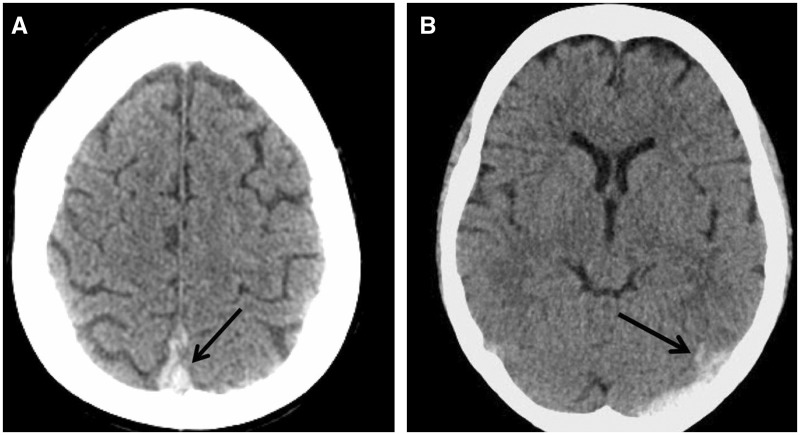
Computed tomography of the head on admission, showing hyperdensity within the superior sagittal sinus (A) and the left transverse sinus (B).
